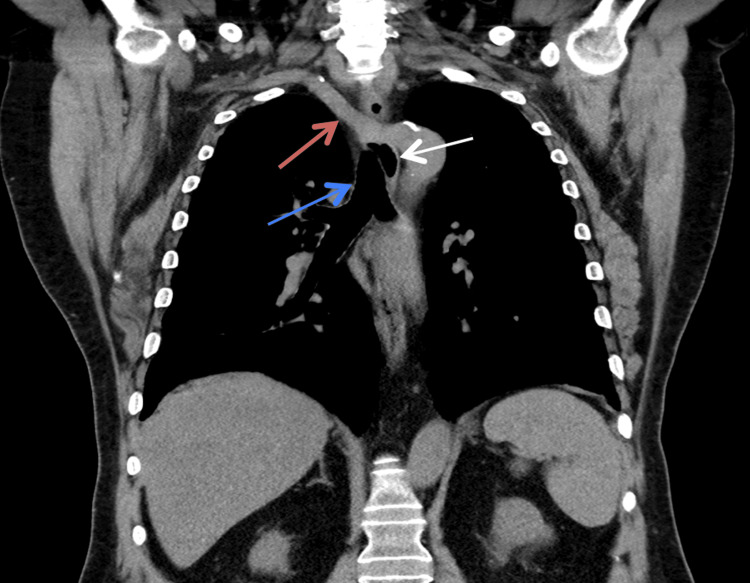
Thoracic CT scan (coronal view) showing the aberrant subclavian artery (red arrow), thoracic esophagus (white arrow), and trachea (blue arrow) CT: computed tomography
